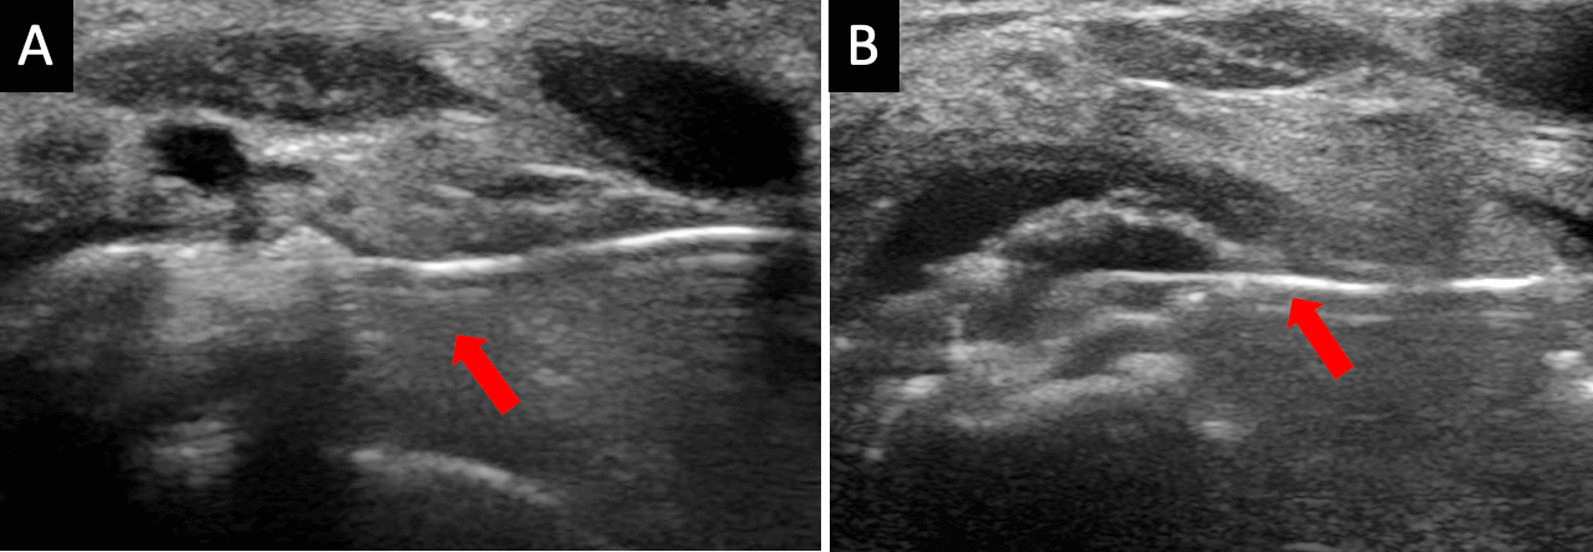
(A), (B) Longitudinal grayscale ultrasound images of the ankle demonstrate ultrasound-guided calcific bursitis lavage using a 20-gauge spinal needle (arrows)
